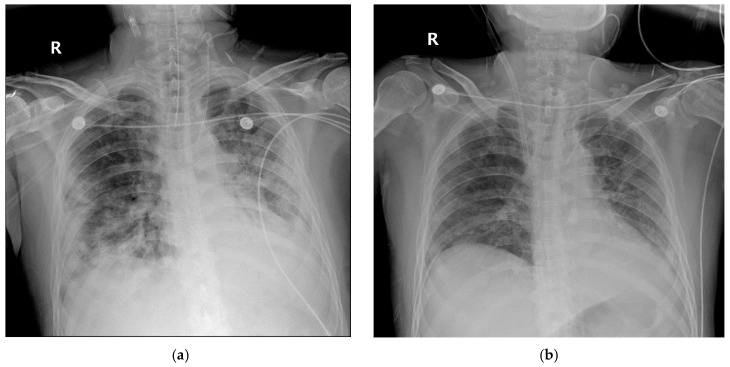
Chest radiography of the patient 3. (a) on the day of ECMO application (b) on the day of ECMO discontinuation.
